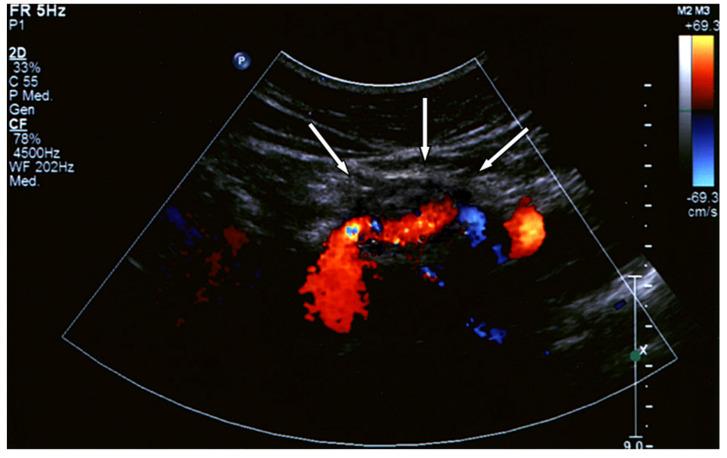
Post-operative CTA following covered stenting of a splenic artery aneurysm.
